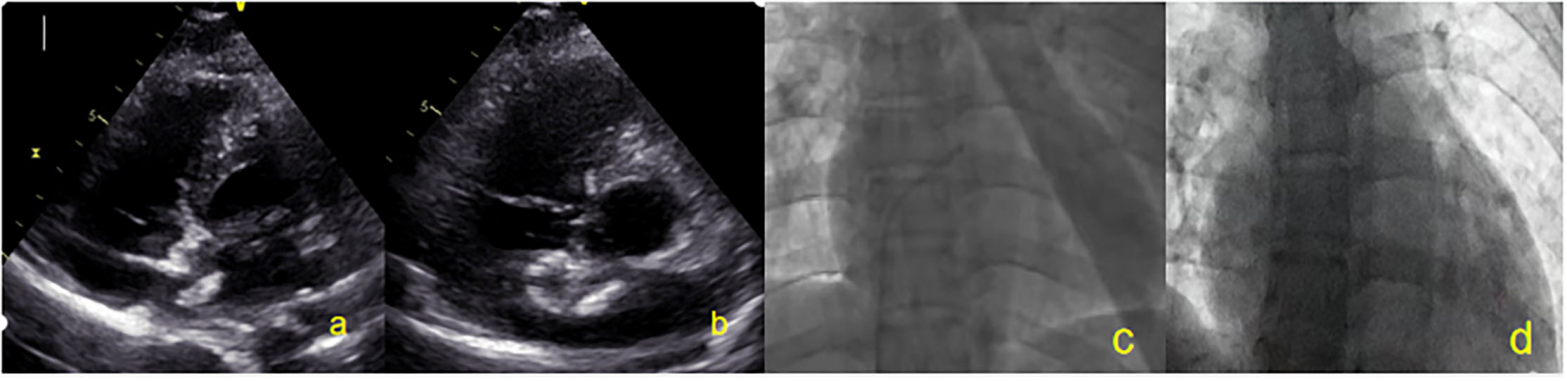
All patients were closured by TTE and DSA. (a) Two-dimensional TTE displaying left disc release. (b) Two-dimensional TTE displaying right disc release. (c) DSA showing the catheter entered the left atrium. (d) After the occluder is released, the invisible occluder is displayed by DSA. TTE, Transthoracic echocardiography; DSA, Digital subtraction angiography.
